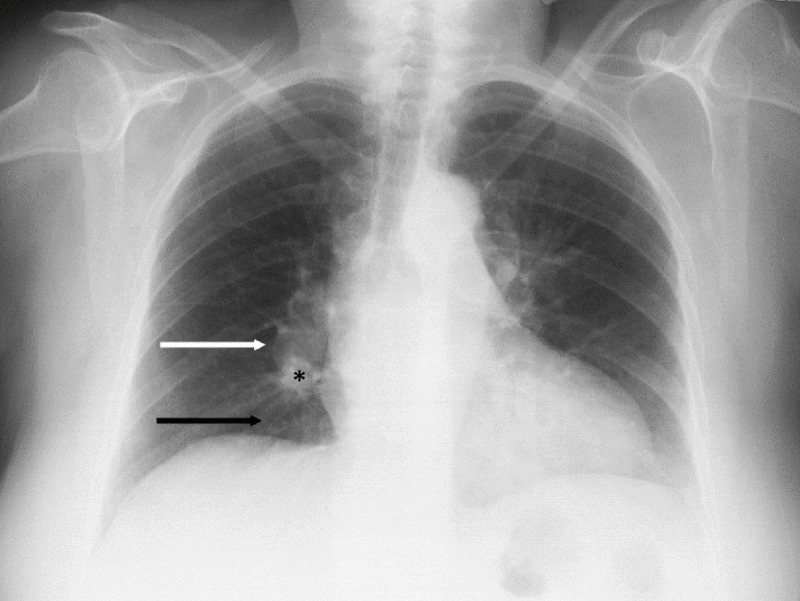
Chest X-ray demonstrating Fleischner’s sign (white arrow), Westermark’s sign (black arrow), and the knuckle sign (black star).
